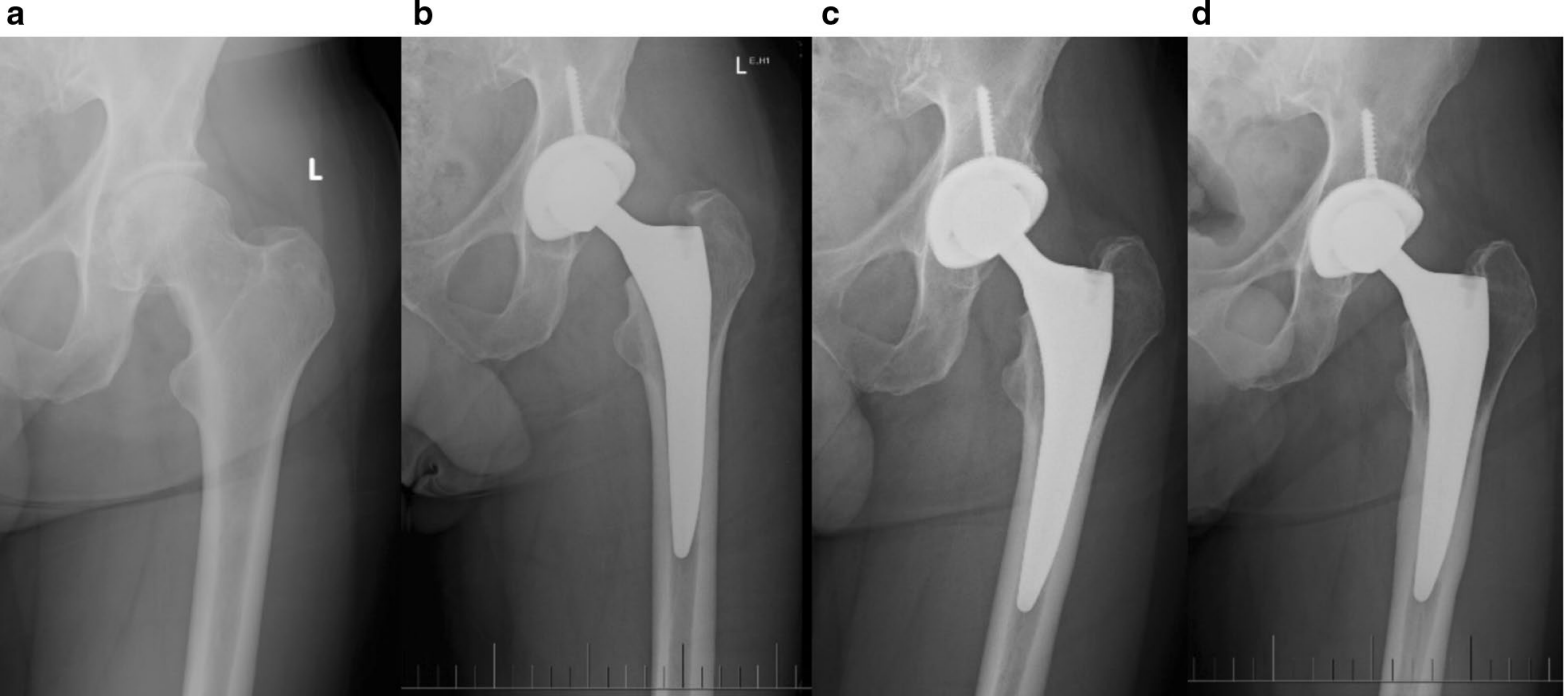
Diaphyseal thickening in 7 out of 47 cases. Example of a 69-year-old male patient with BMI of 25.7 kg/m^2^. Follow-up X-rays: a pre-OP, b 6 weeks, c 1 year, d 4 years
